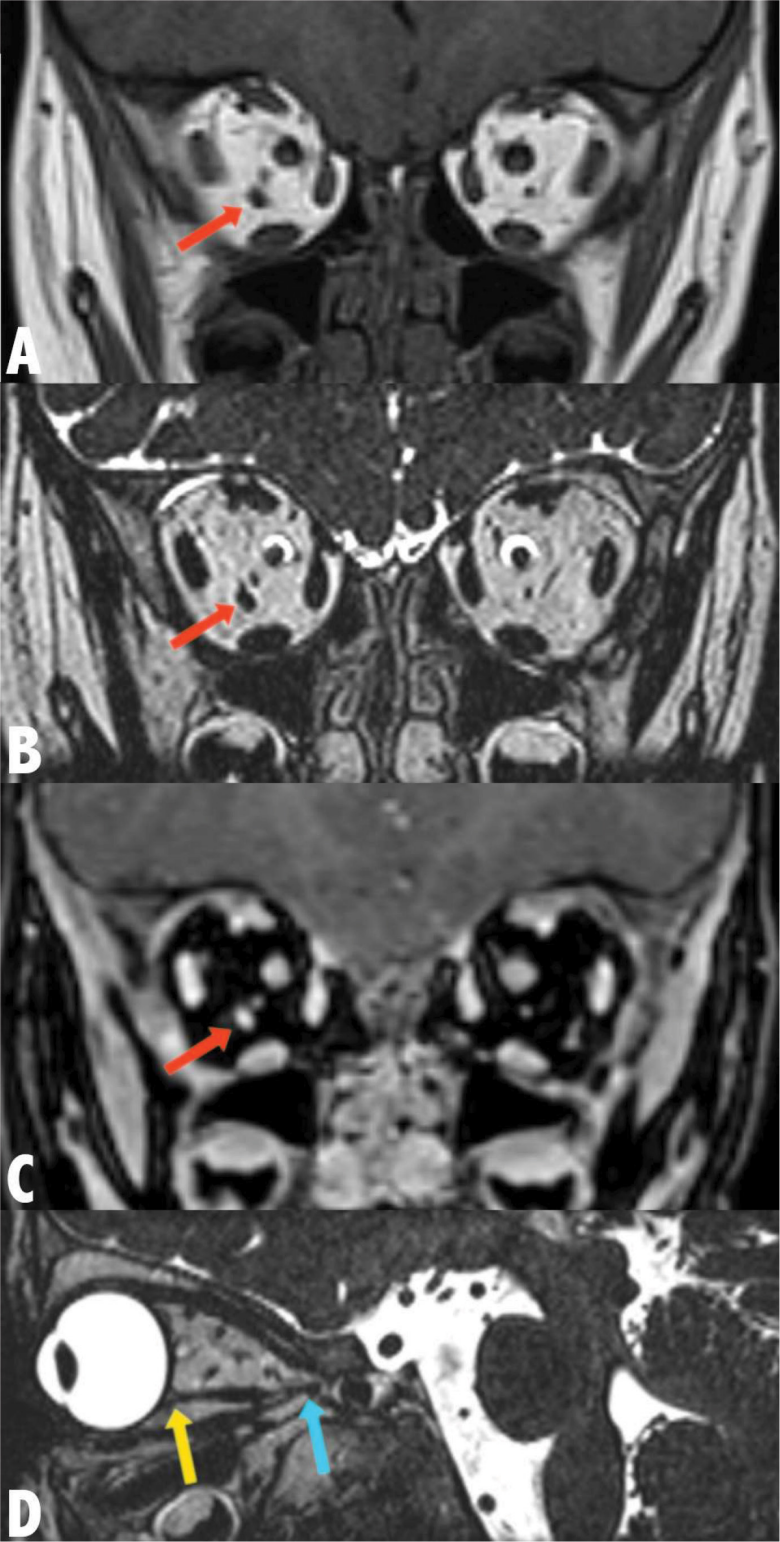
MRI Coronal T1‑WI (a) and coronal T2‑WI (b) of the orbits demonstrate an accessory extraocular muscle in the right orbit (red arrow), appearing isointense to other extraocular muscles. Coronal post‑gadolinium T1‑WI with fat saturation (c) reveals the accessory extraocular muscle (red arrow) with increased signal intensity, consistent with other extraocular muscles. Sagittal T2‑WI (d) of the right orbit shows the accessory extraocular muscle attaching anteriorly to the posterolateral aspect of the globe (yellow arrow) and posteriorly the annulus of Zinn (blue arrow).
